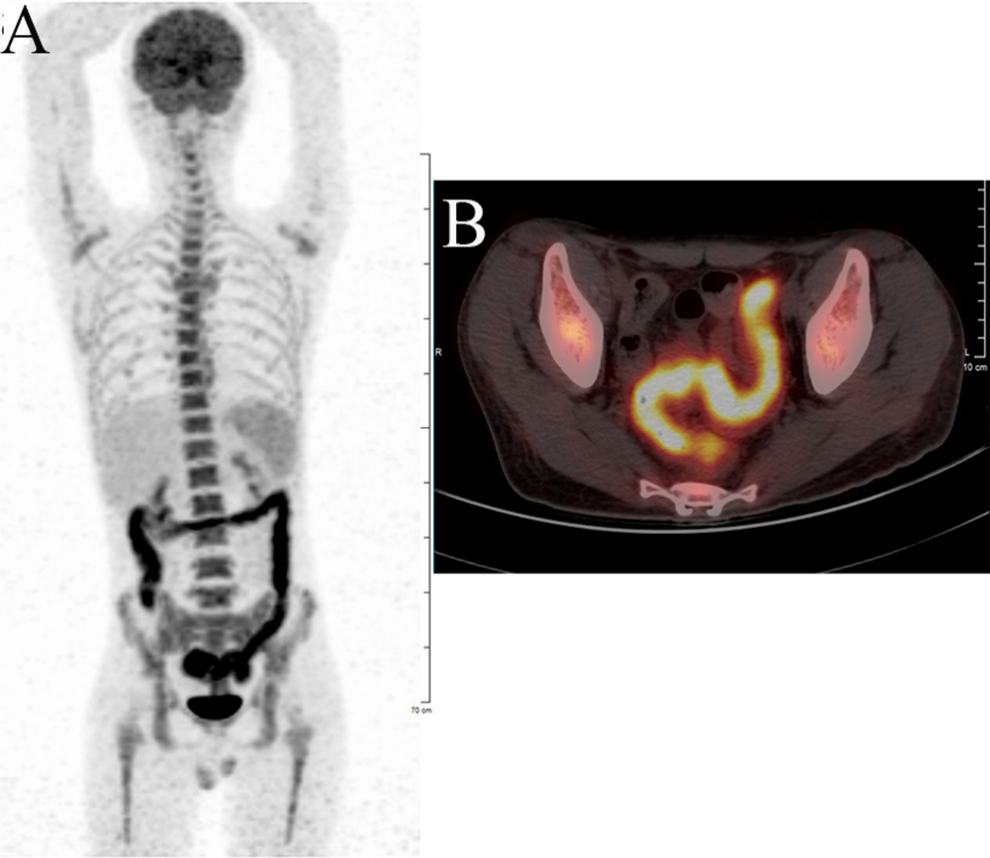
F-18 fluorodeoxyglucose positron emission tomography with computed tomography (^18^F-FDG PET/CT) showing significant increased FDG uptake in the intestinal wall of the whole colon. (A) Body maximum intensity projection (MIP) image. (B) abdominal PET/CT.
